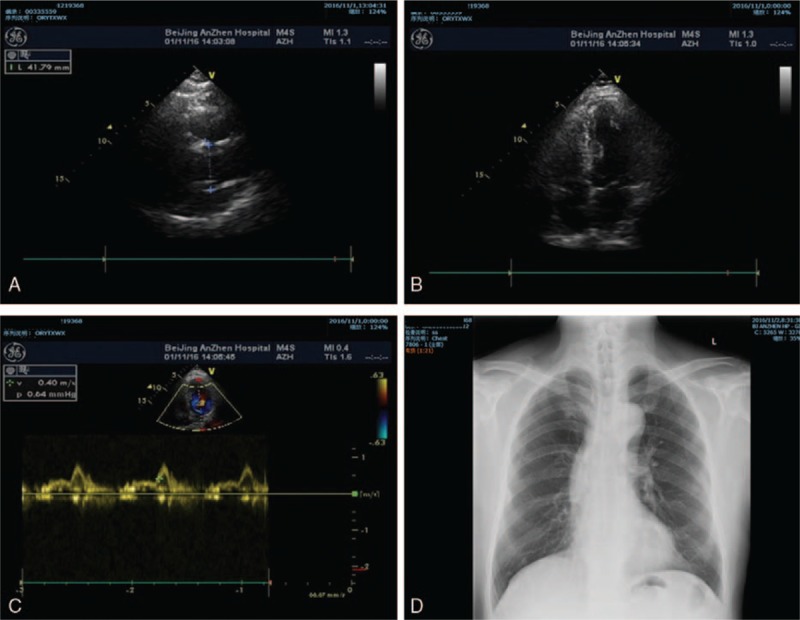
(A–C) Preoperative Doppler ultrasound heart (UCG) results, demonstrating acceptable cardiac function, with a left ventricular ejection fraction (LVEF) of 52% and no valvular regurgitation or hydropericardium. (D) Preoperative chest X-ray, no abnormality could be found in the imaging examination.
